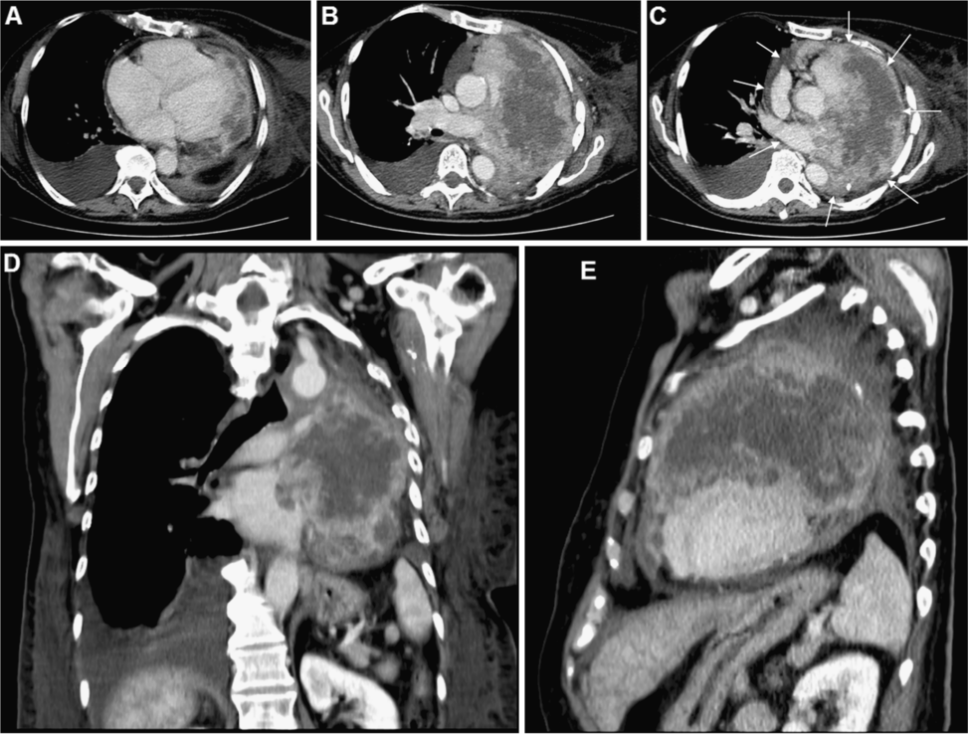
Radiological evaluation. Contrast-enhanced axial (Figures 3A-3C), coronal (Figure 3D) and sagittal (Figure 3E) multislice computed tomography scan of the chest revealed massive, predominantly necrotic metastatic tumour mass (13×10 cm in size on axial view) with pericardial and heart involvement (arrows). The tumour was partially necrotic and infiltrated the thoracic aorta and the pulmonary trunk with extension into the left atrium.
